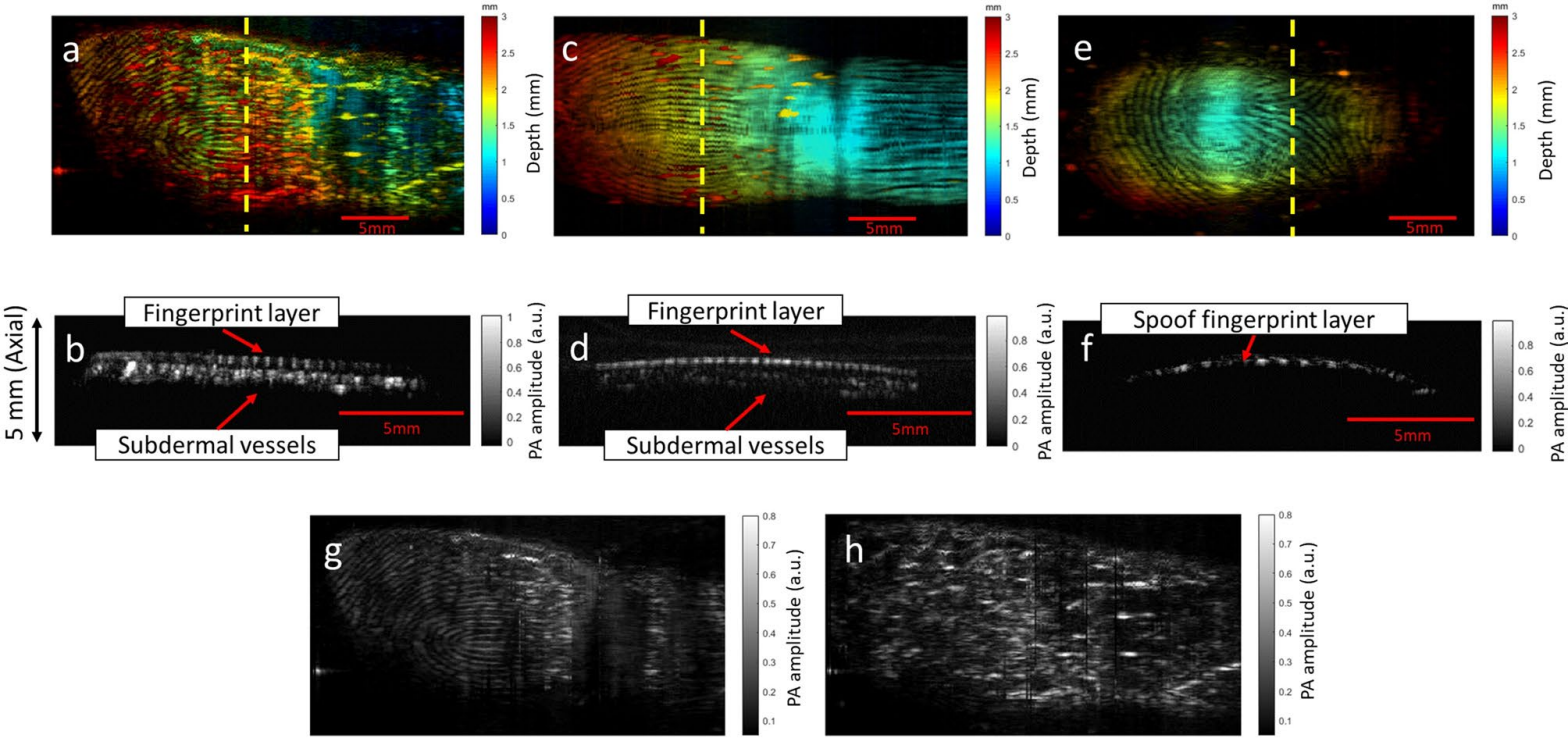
PAT results of human fingerprint. (a) Depth-encoded MAP image of the fingerprint obtained with ultrasound gel coupling. (b) Cross-sectional PAT image across the yellow dashed line in (a). (c) Depth-encoded MAP image of the fingerprint obtained without ultrasound gel coupling. (d) Cross-sectional PAT image across the yellow dashed line in (c). (e) Depth-encoded MAP image of the spoof fingerprint obtained with ultrasound gel coupling. (f) Cross-sectional PAT image across the yellow dashed line in (e). (g) Extracted fingerprint MAP image with ultrasound gel coupling. (h) Extracted subdermal vasculature MAP image with ultrasound gel coupling.
